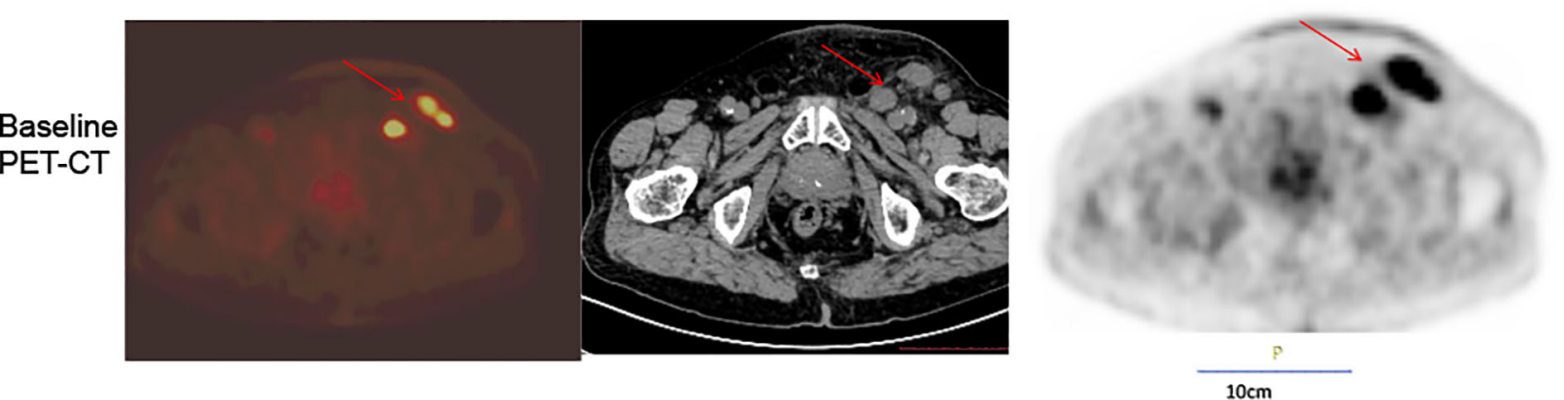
Baseline PET-CT.Multiple enlarged lymph nodes were noted adjacent to the left external iliac vessels in the inguinal region and with increased FDG avidity by PET-CT scan.
